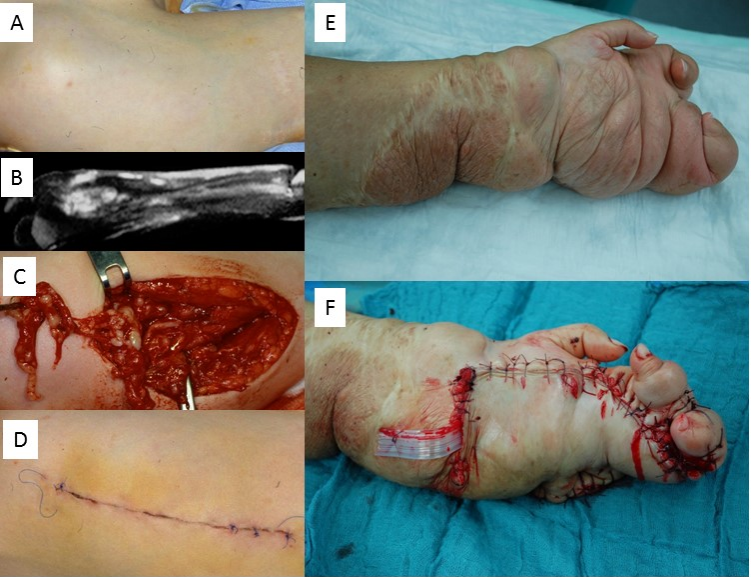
A–D. Resection of plexiform neurofibroma of the right upper extremity in a patient with NF1, who was very sensitive to pain in this area. A. In this case, the effect of the invasive tumor on the skin is hardly visible. Palpation shows only the superficial portions of the nodular tumors (“bag of worms”). Skin pigmentation in this area is hardly changed. B. Magnetic resonance image indicates a nodular tumor and hyperintense skin covering the tumor-affected region. C. Intraoperative situs reveals a dense conglomerate of enlarged and tumorous peripheral nerves. D. Wound closure by primary intention. E. Female patient with NF1 and history of multiple surgical interventions of the left upper extremity. F. Debulking in the palm region was carried out in order to improve gripping function of the remaining fingers.
